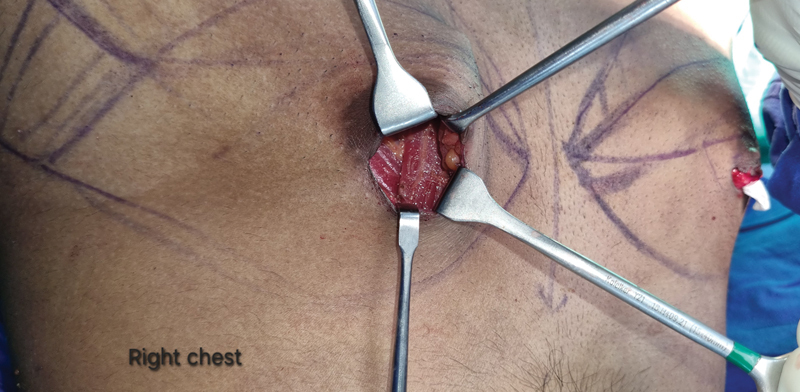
Vertical muscle fibers: right parasternal region, anterior to the pectoralis major muscle fibers.
